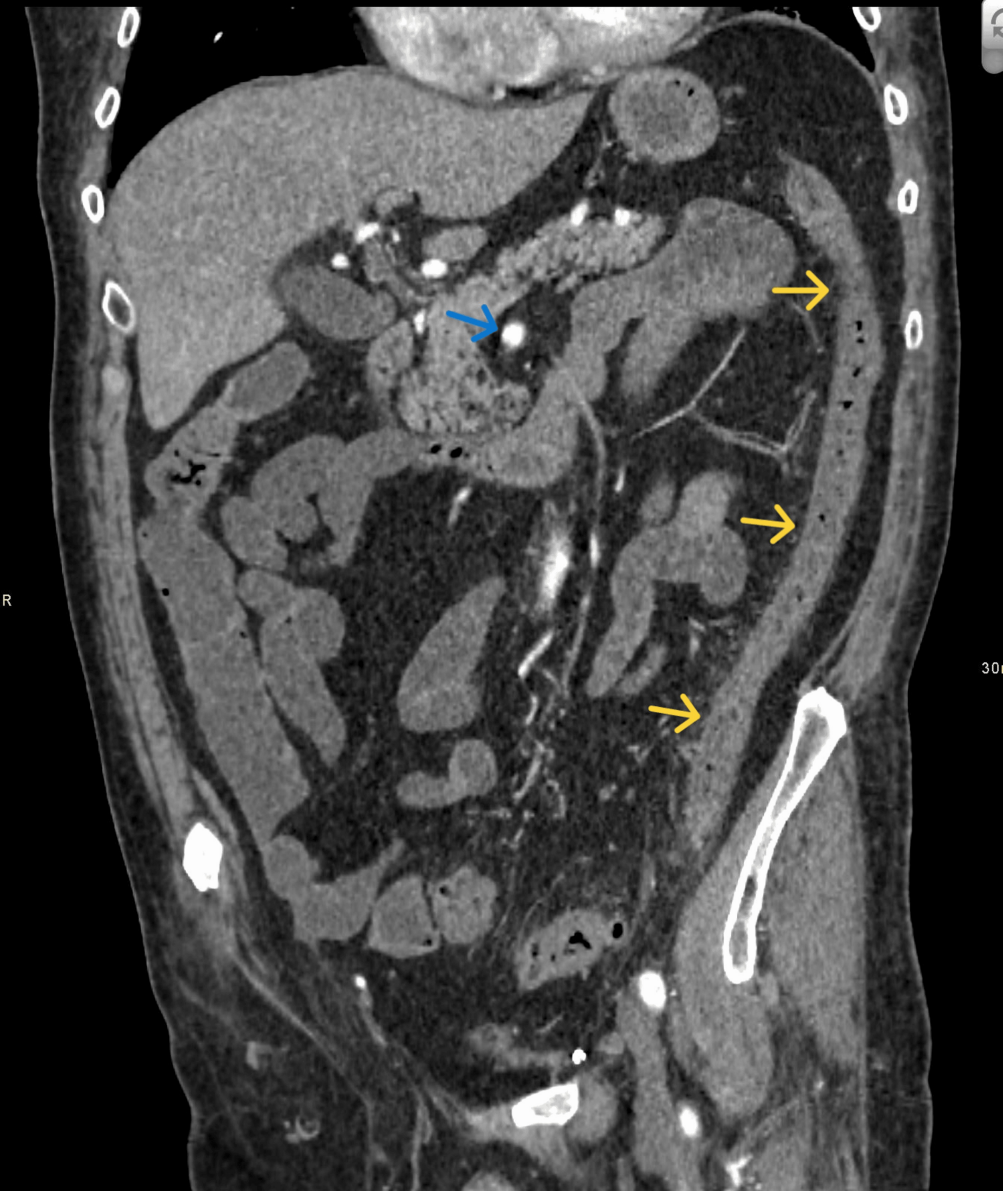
Selected coronal reconstruction image of a CT abdomen and pelvis study in the arterial phase demonstrating 'circumferential wall and fat stranding' (yellow arrows). Note that the superior mesenteric artery (SMA) is patent and shows homogeneous opacification (blue arrow). Blue Arrow: Superior mesenteric artery Yellow Arrows: Inflamed left colon. Blue Arrow: Superior mesenteric artery
